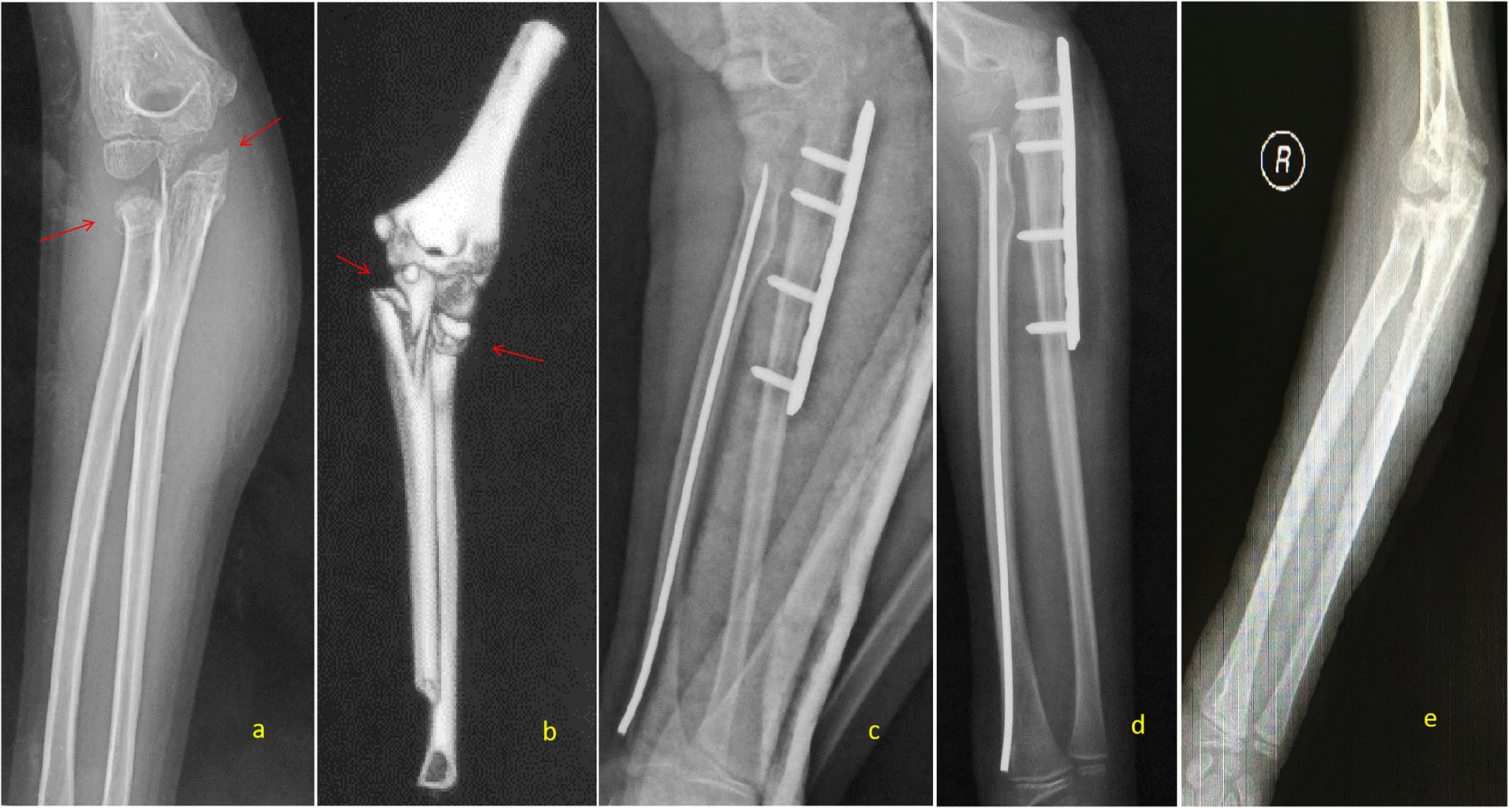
A 9-year-old boy classified type IV injury in right elbow. a A-P view of X-ray film show fracture in coronal plane of olecranon with displacement less than two thirds of ulna (arrow) associated with radial neck fracture with angulation less than 30°. b 3D reconstructed image of CT scan show the precise injury complex. c A-P view of X-ray film shows this boy was treated with open reduction and internal fixation with locking-plate for olecranon and close reduction and internal fixation with ESIN for radial neck; the broad of olecranon in coronal plane was corrected. d Three months postoperation, A-P view of X-ray film show the olecranon and radial neck fracture healed without malunion. e Six months postoperation, A-P view of X-ray film shows no radial head subluxation could be observed after the ESIN and plate removed; the flexion, extension, pronation, and supination were normal.
